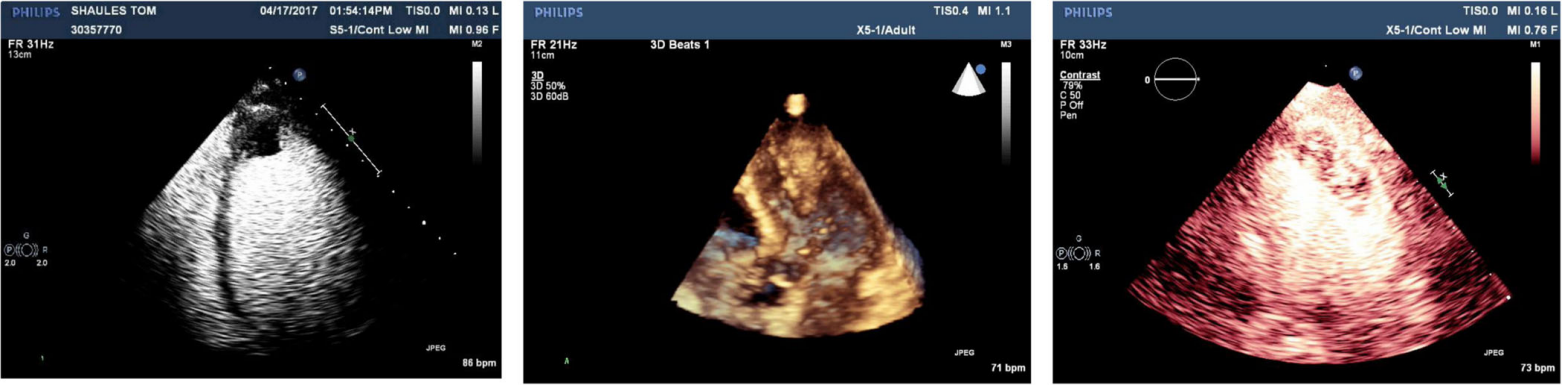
Left panel delineates an apical thrombus that is free of any ultrasound reflectances. Middle panel show the echo of a patient with a large apical tumor that contains reflectances generated by tissue in homogeneity. Right panel demonstrates the same tumor after contrast agent administration which clearly delineates the persistent vasculature.
